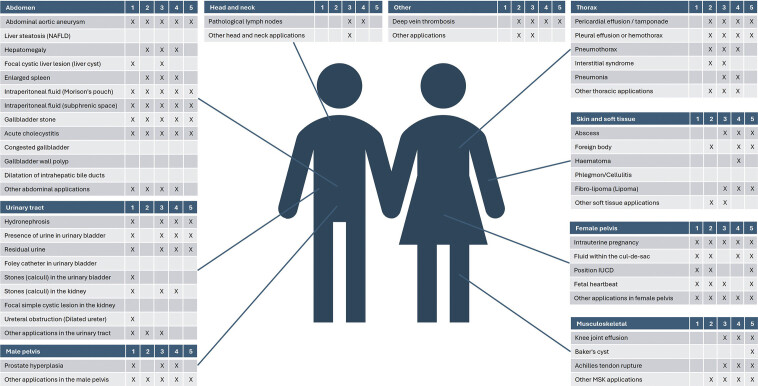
Comparing results to previous Delphi studies. Ultrasound applications included in this Delphi study compared to applications included in previous Delphi studies: 1 = Camard et al. France 2024 (reference 23), 2 = Mans et al. South Africa 2023 (reference 24), 3 = Conangla-Ferrin et al. Spain 2022 (reference 22), 4 = Homar et al. Slovenia 2020 (reference 21), and 5 = Løkkegaard et al. Scandinavian countries 2019 (reference 18).
